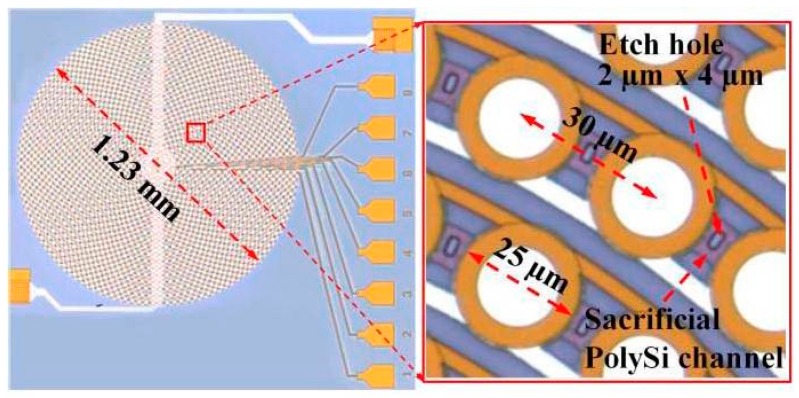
High fill-factor annular array for high frequency piezoelectric micromachined ultrasonic transducers (PMUTs), optical image of the PMUTs array (Left) and close-up picture of individual 25 μm diameter PMUTs (right). Left: The eight channels are connected to individual bond-pads through the top-electrode metal and a single bond-pad is connected to the common bottom electrode. Right: The sacrificial poly-Si is removed via 2 μm by 4 μm etch holes between each PMUT. The rings of PMUTs are connected through the top-electrode metal layer (dark gold) and bottom electrode (white) (Reproduced with permission from IEEE [35]).
